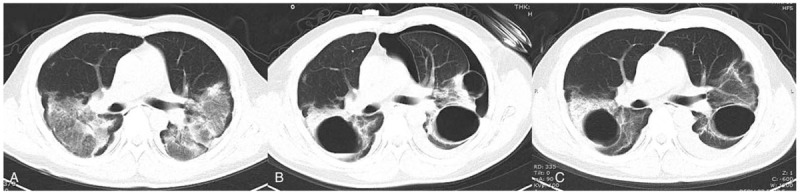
A 38-yr-old man diagnosed with severe COVID-19 pneumonia. (A) The primary CT scan showed bilateral, patchy, ground-glass opacities with co-existing consolidations. (B) Nine days later, a follow-up CT scan showed multiple cysts in the ground-glass opacities (bilaterally) and a ∼20% left pneumothorax. The cysts featured a smooth inner wall; the maximum diameter of these cysts was about 5 cm. (C) The third CT scan (performed 5 d after the second CT scan) showed that the left pneumothorax and a small cyst on the left pulmonary margin had disappeared; the remaining cysts reduced in size slightly.
